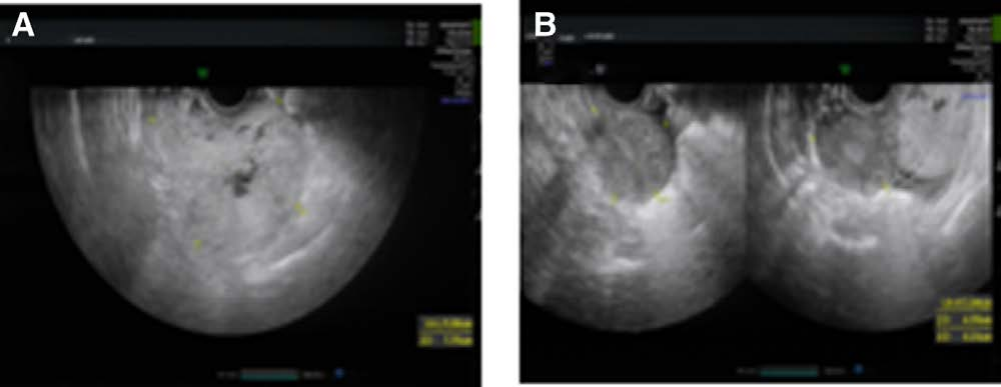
Ultrasonography: (A) a hyperechoic nodule with an indistinct boundary; (B) the nodule was connected to the uterus with an irregular shape.
